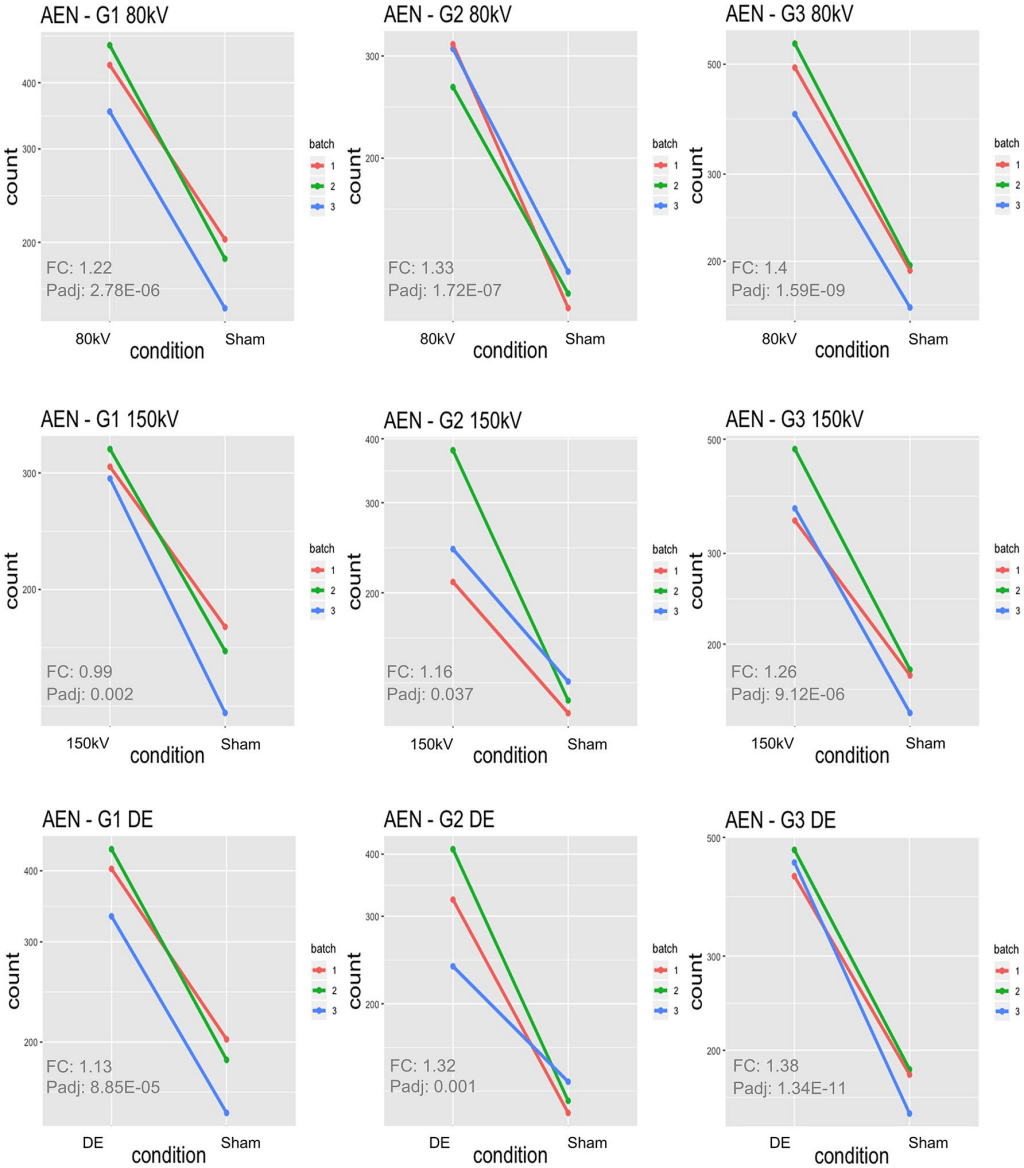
Gene expression plots for *AEN* for 80 kV single-energy CT (SECT), 150 kV SECT and dual-energy CT (DE) irradiation: Each plot depicts gene counts obtained from RNA sequencing data of the respective proband (G1-3) with post-irradiation gene count displayed to the left and corresponding sham-irradiated gene counts displayed to the right. Colored lines (green, blue and red) indicate the three replicate experiments.
